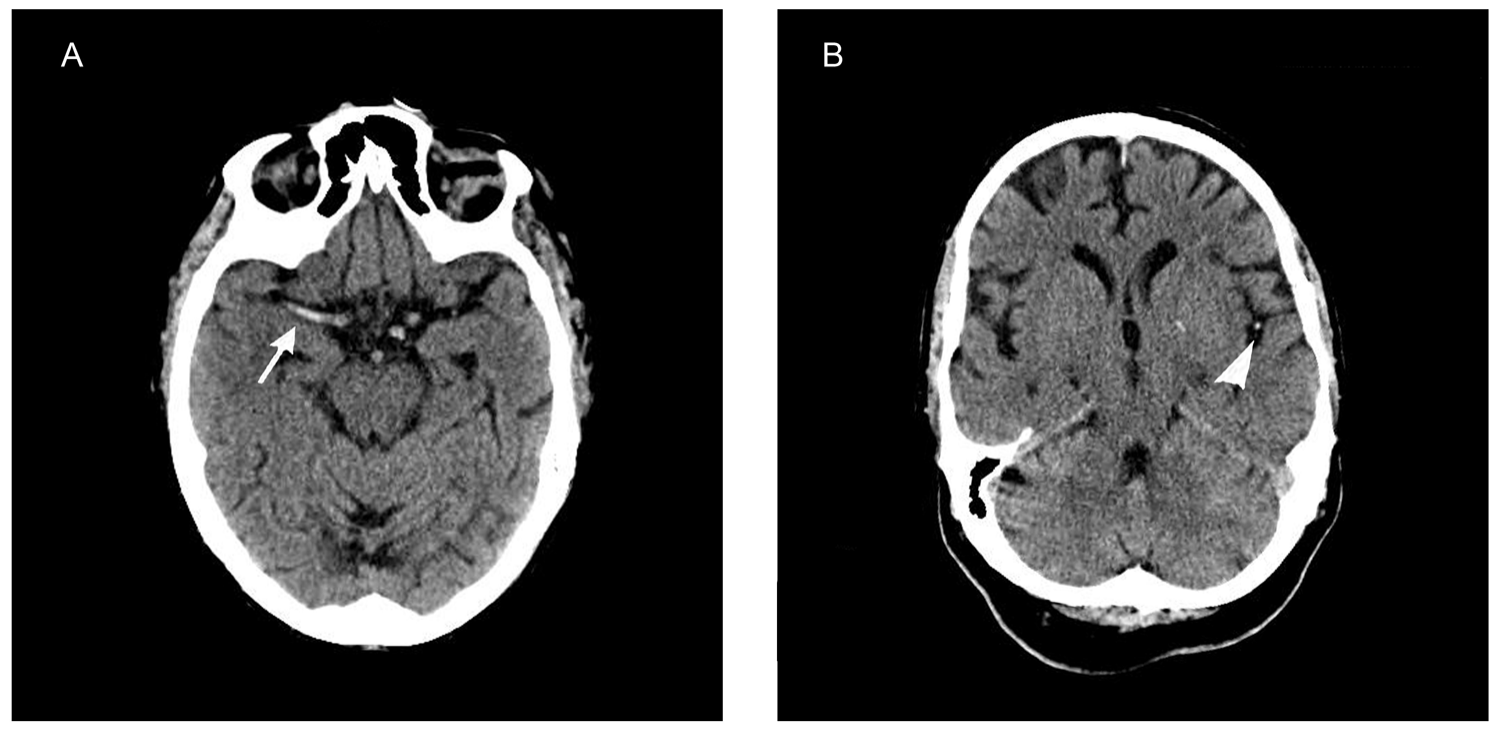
Hyperdense artery sign on noncontrast CT. (A) Transverse CT scan showing a proximal hyperdense artery sign (arrow) in the M1 segment of MCA. (B) Noncontrast CT showing a distal hyperdense artery sign in the sylvian fissure (arrowhead), which was also referred to as the MCA dot sign.
